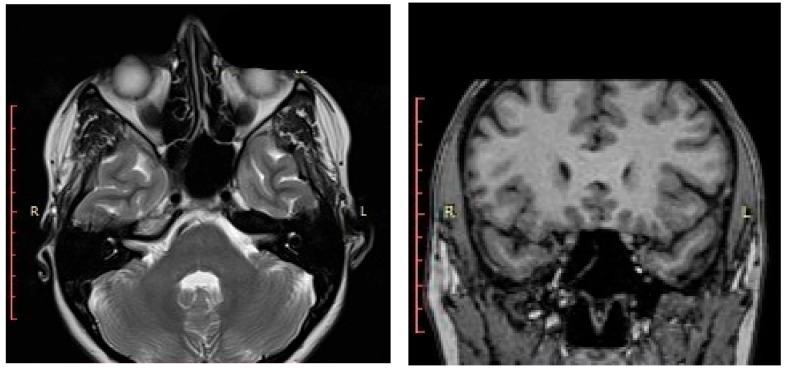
Enhanced brain MRI (axial and coronal plane)—no lesion detected within the left sphenoid sinus four months postoperatively.
